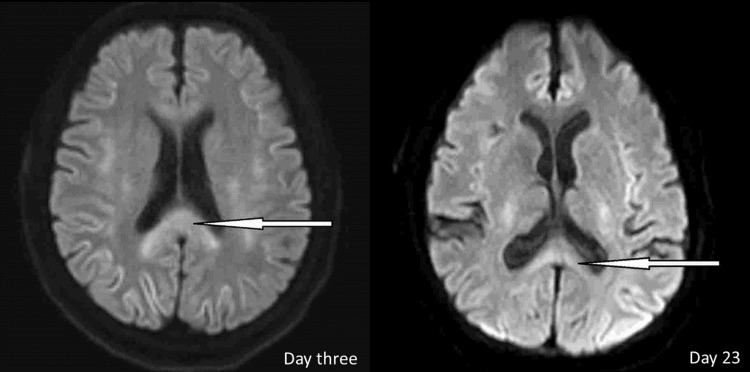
MRI of the brain obtained three days after symptom onset revealed significant diffusion restriction along the corpus callosum, which markedly improved on repeat MRI of the brain three weeks later
